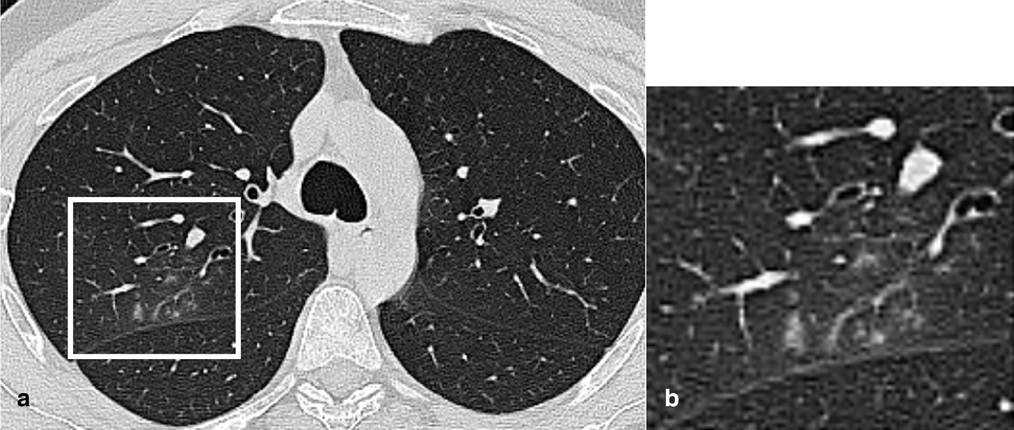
Axial CT image of a 25-year-old male Covid-19 patient (γ variant) showed perifissural small centrilobular nodules connected to linear branching opacities (white box), in right upper lobe.
